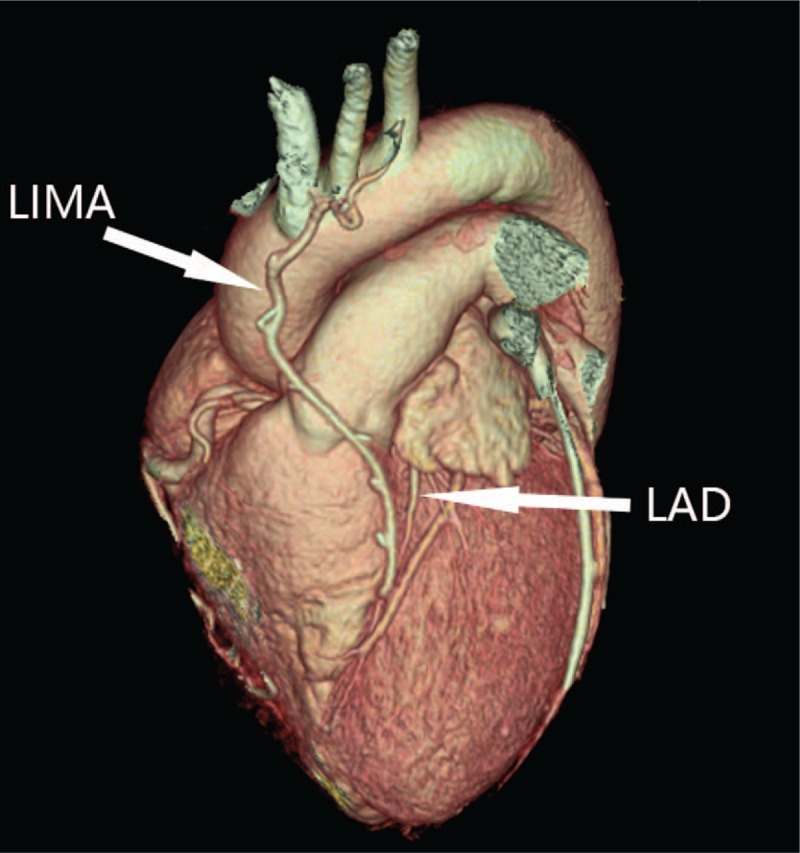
Three-month follow-up CT coronary angiography showed patent LIMA-LAD graft with the small LAD. CT = computed tomography, LAD = left anterior descending, LIMA = left internal mammary artery.
